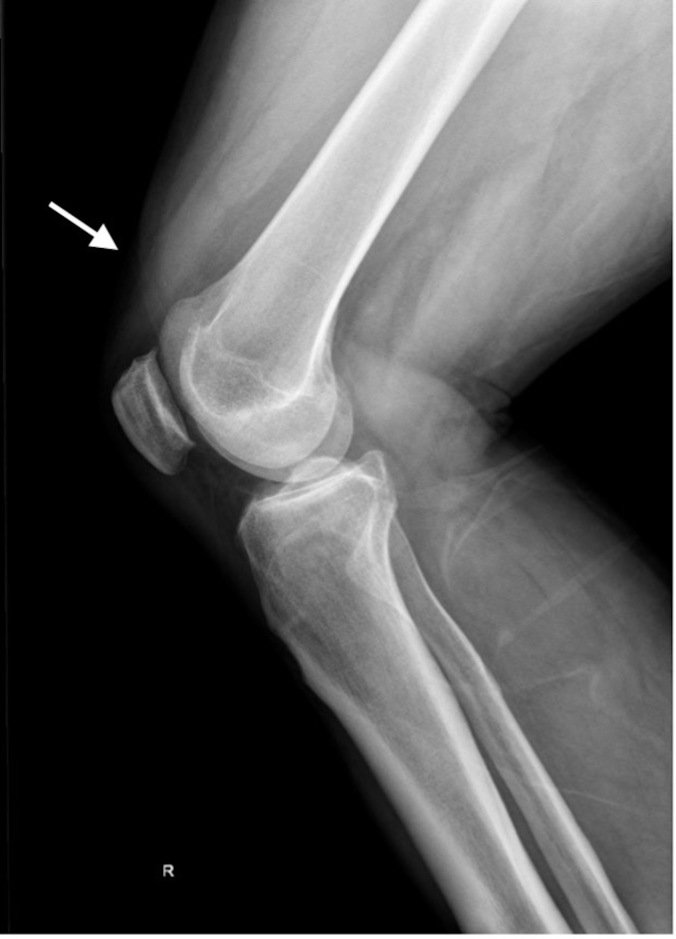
Plain radiograph of the right knee demonstrating mild suprapatellar soft tissue swelling (arrow).
